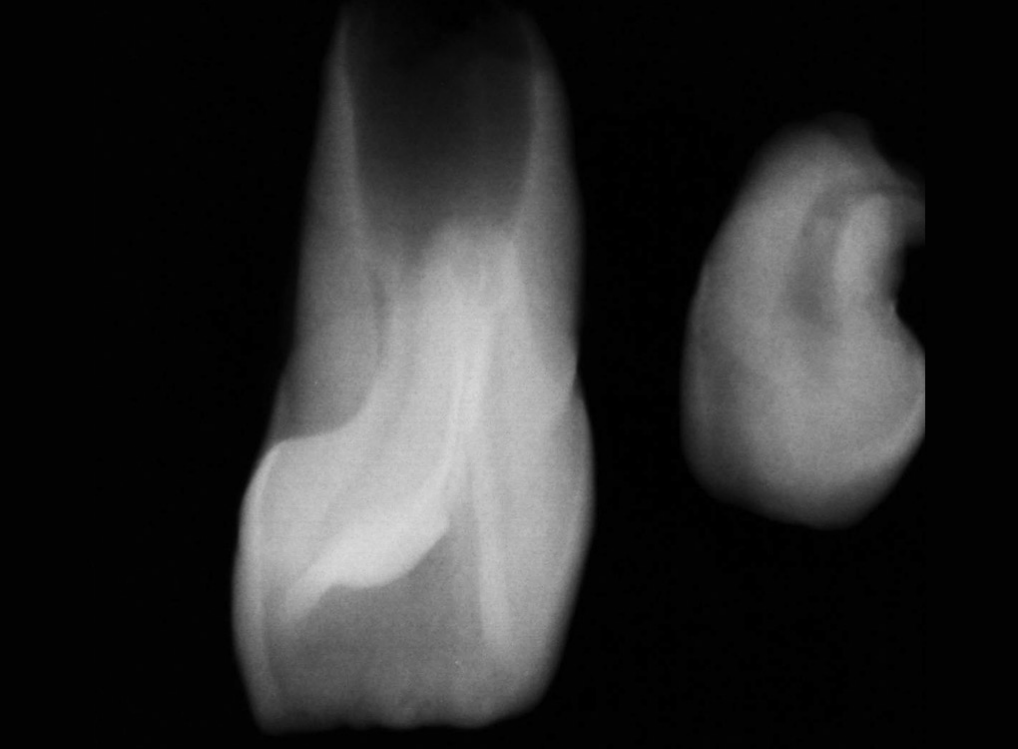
Radiograph of supernumerary teeth depicting invagination in the tuberculate type
